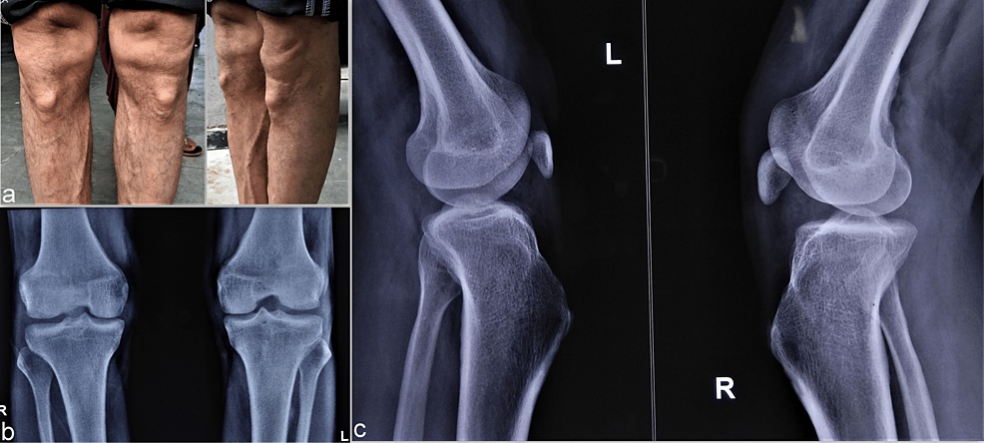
Knee changes in Case 2 The clinical image showing a depressed region at the location of the bilateral patella and a knobby lesion below it, corresponding to bilateral tibial tuberosities (a). The radiograph corresponds to the bilateral hypoplastic patellae (b) and associated hypertrophied bilateral tibial tuberosity (c).
